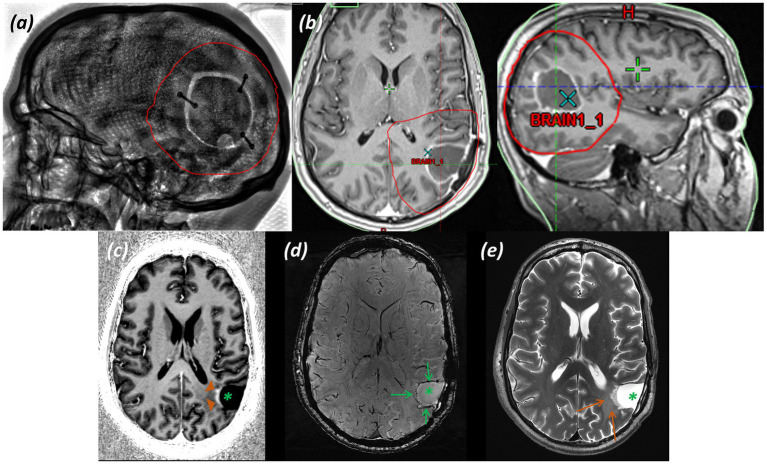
Patient 6: Glioblastoma, IDH-wildtype (CNS WHO grade 4), post-operative and post-radiotherapy. (a) CT image displaying craniotomy metalware, (b) Radiotherapy planning maps in axial and sagittal planes, with PTV (red) marked, and (c) T_1_-weighted MP2RAGE UNI image post-gadolinium contrast, (d) SWI, and (e) T_2_-weighted TSE image through the level of the resection cavity. (Green asterisk* indicates resection cavity; green arrow indicates blood degradation products surrounding the resection cavity; orange arrowhead indicates post-contrast enhancement; orange arrow indicates abnormal white matter).
